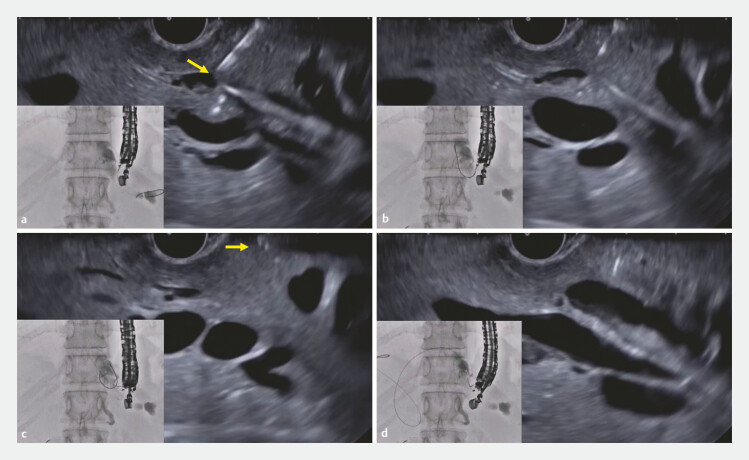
Endoscopic ultrasound images.
